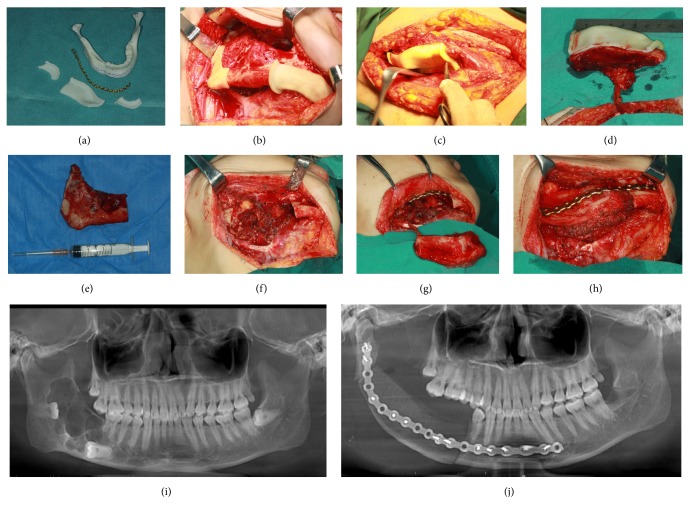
Procedures for the SGT (simple guiding templates). (a) Overview of the cutting guides, prebending titanium plate and reconstructed model. (b) Application of the simple cutting guides onto the surface of mandible, on both side of the lesion. (c) Application of the cutting guide on the iliac area. (d) Harvest of the vascularized iliac bone before pedicle division. (e) The removed mandibular lesion according to the cutting guide. (f) The mandibular defect after mandibulectomy. (g) Restoration of the mandibular continuity by prebending titanium plate and the deep circumflex iliac artery and vein of the bone flap was anastomosed with facial artery and vein. (h) The iliac bone was reshaped and put into the defect and fixed: (i) presurgery panoramic radiograph showed lesion in the right gonion and (j) postsurgery panoramic radiograph showed the lesion was removed and embed by iliac bone.
